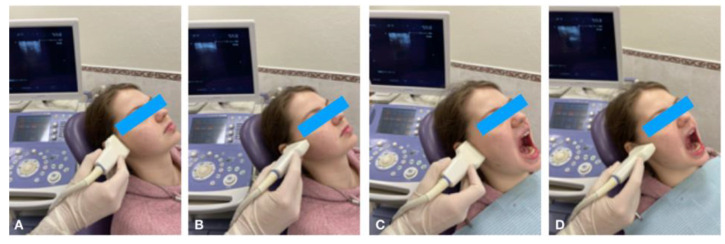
Ultrasonography of TMJ in longitudinal (A) and transverse (B) scans in the closed mouth position; longitudinal (C) and transverse (D) scans in the open mouth position.
